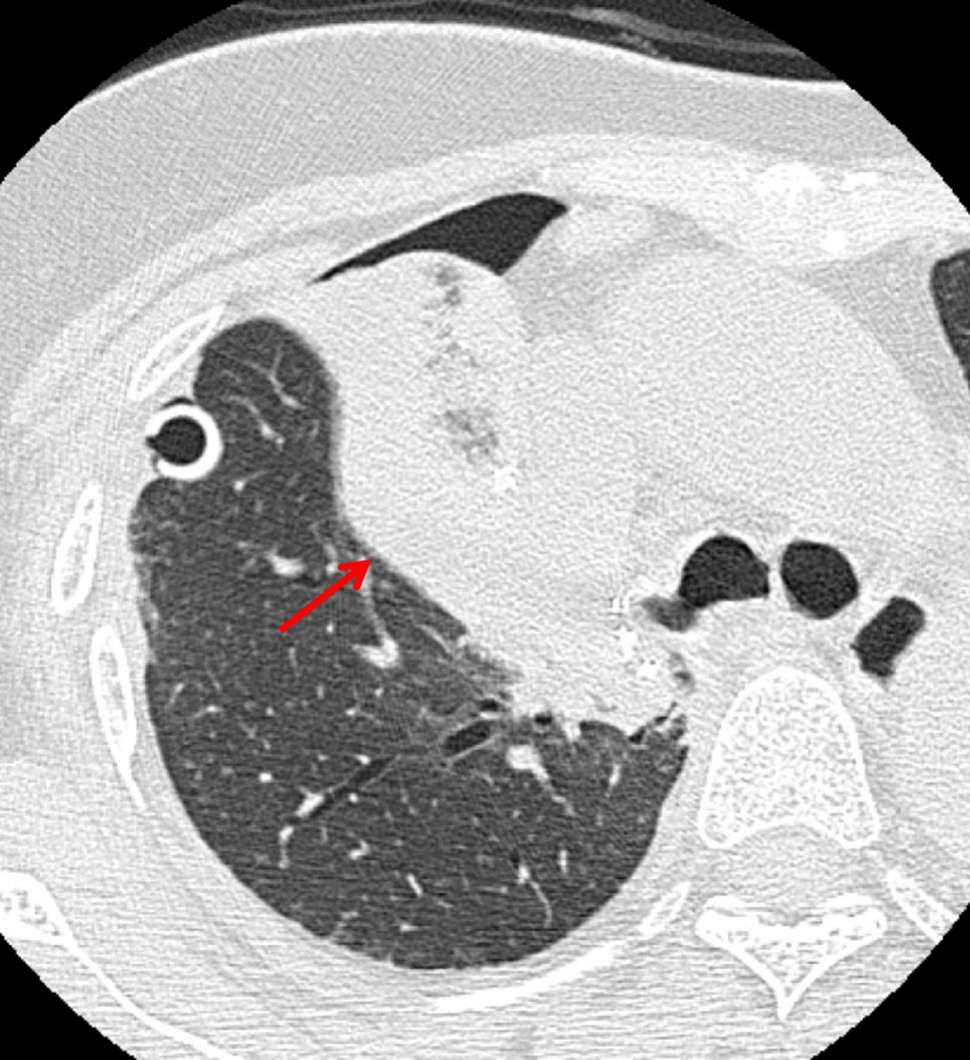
Lung torsion following right upper lobectomy. CT performed 2 days postoperatively shows consolidation in the twisted middle lobe (arrow)
